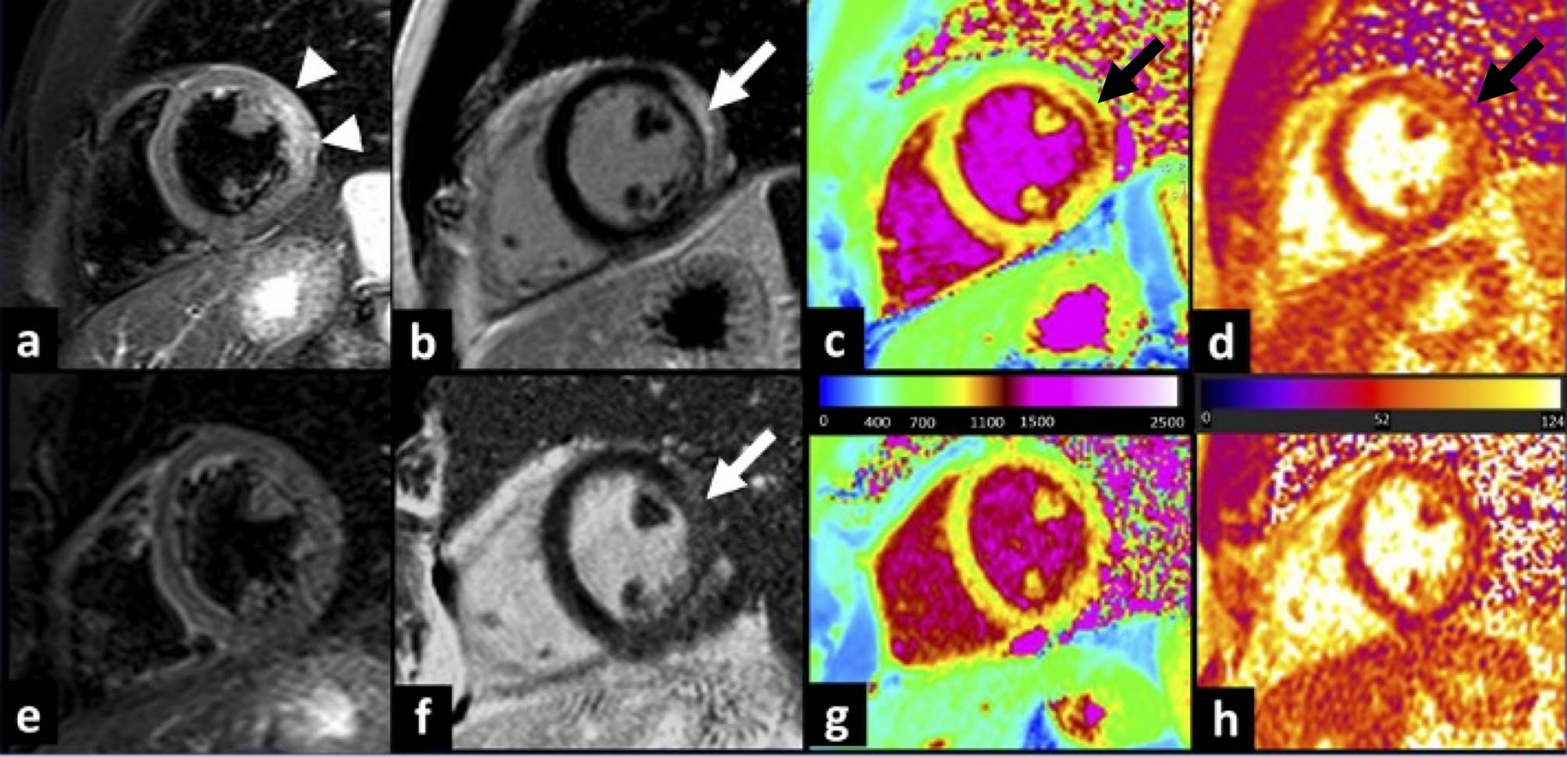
Vaccine-associated myocarditis. T2-weighted images (a) revealed the presence of myocardial edema located in the lateral wall of the left ventricle (arrowhead). A subepicardial LGE stria was evidenced on the same myocardial segments (b, white arrow). Those findings were confirmed by increased T1 (c) and T2 (d) mapping values on the lateral wall. At 117 days follow-up, CMR revealed a resolution of myocardial edema (e), with a reduction in LGE extension (f). Mapping sequences showed a decrease in T1 mapping values (g) and T2 values (h) that returned within the normal range
